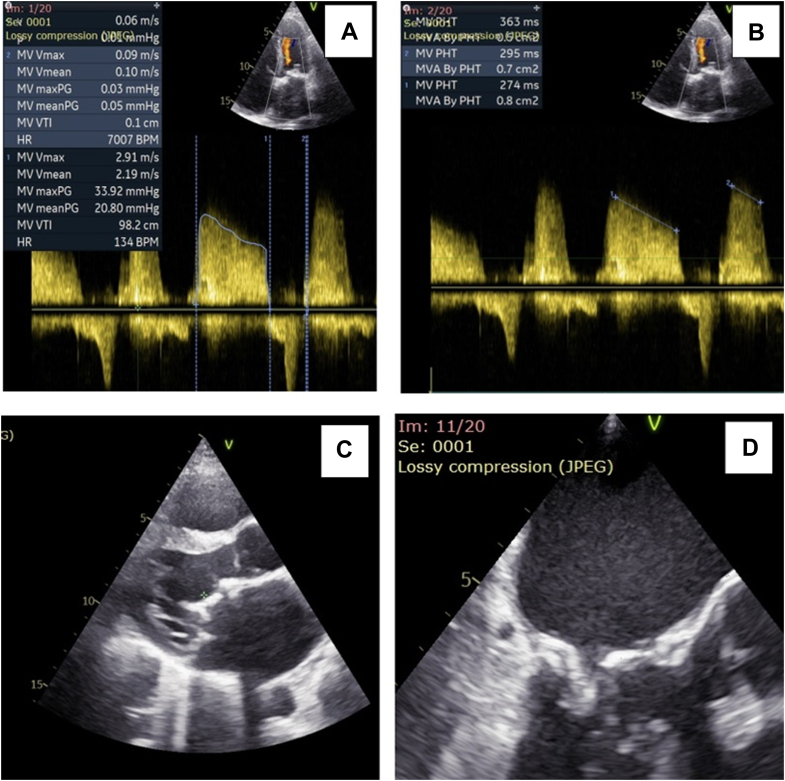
TTE and TEE Assessment of Degenerated Bioprosthetic Mitral Valve (A) Apical 4-chamber view on TTE and continuous-wave Doppler of mitral valve inflow showing a mean pressure gradient of 20 mm Hg. (B) Pressure half-time in apical 4-chamber view on TTE showing an average mitral valve area of 0.7 cm^2^. (C) TEE parasternal long-axis view showing degenerated bioprosthesis in the mitral position. (D) TEE 3-chamber view at 150° angle showing degenerated bioprosthesis in the mitral position. TEE = transesophageal echocardiography; TTE = transthoracic echocardiography.
